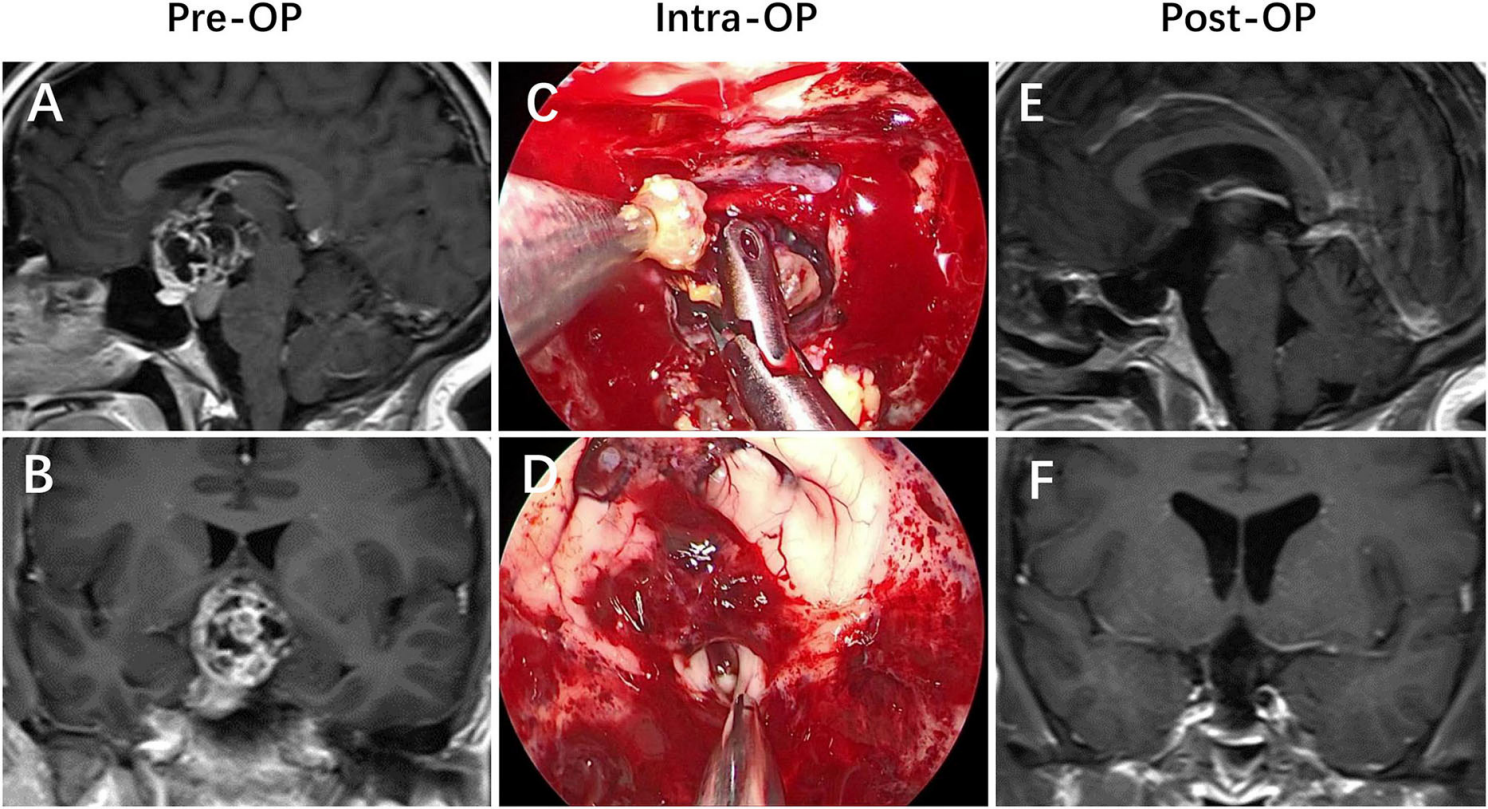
Craniopharyngioma with hypothalamic invasion. (A,B) Preoperative enhanced MRI demonstrated that an extraintraventricular mass developed from the pituitary gland and/or the pituitary stalk that had invaded the third ventricle after breaking through the third ventricle floor. (C,D) Intraoperative views revealed that the patient achieved a gross-total resection of the lesion *via* the endonasal endoscopic endonasal infrachiasmatic corridor. (E,F) Postoperative enhanced MRI revealed that the lesion was totally removed, with the TVF opened.
